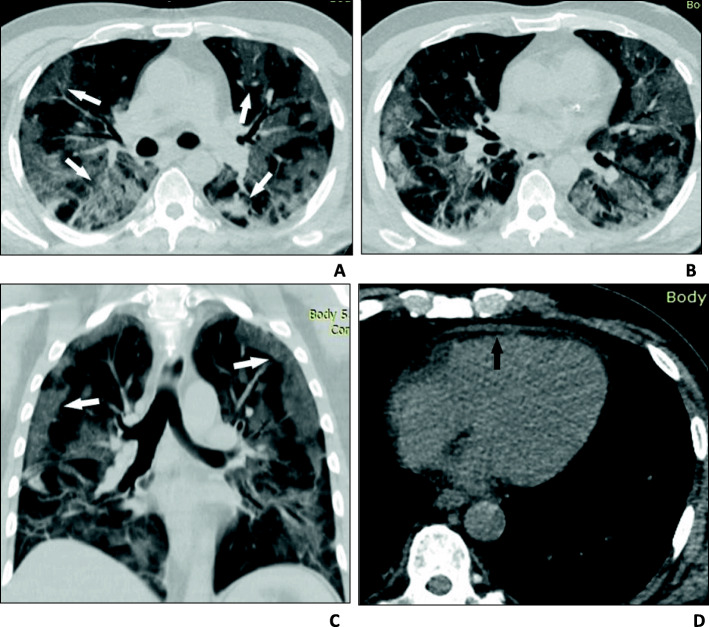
A 60-year-old man who presented with fever and cough followed by centrithoracic chest pain that improved on leaning forward and increased when lying supine and progressive dyspnea requiring admission to the intensive care unit. His PaO_2_/FiO_2_ ratio was 220 mmHg. a–c Axial and coronal thin-section unenhanced CT scan shows diffuse bilateral confluent and patchy ground-glass and consolidative (white arrows) pulmonary opacities with peripheral and central distribution. d Axial image shows thickened pericardium > 2 mm (black arrow) suggesting pericarditis confirmed by echocardiography
